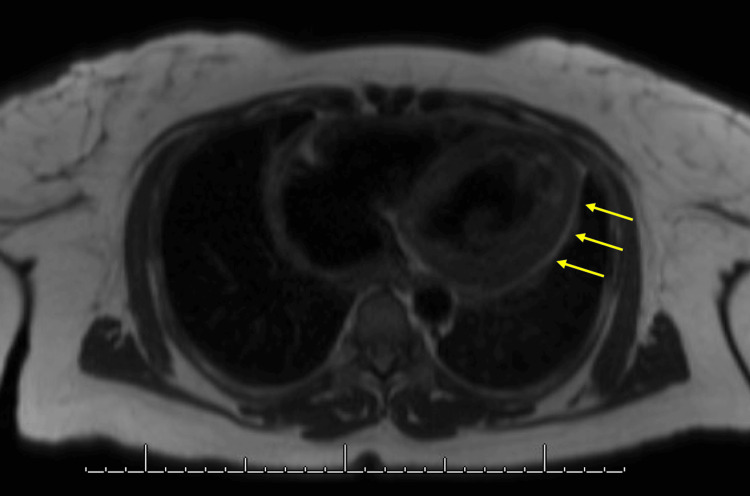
Post-hospitalization cardiac MRI Cardiac MRI taken following discharge from the hospital. The yellow arrows highlight the thickening of the pericardium.
